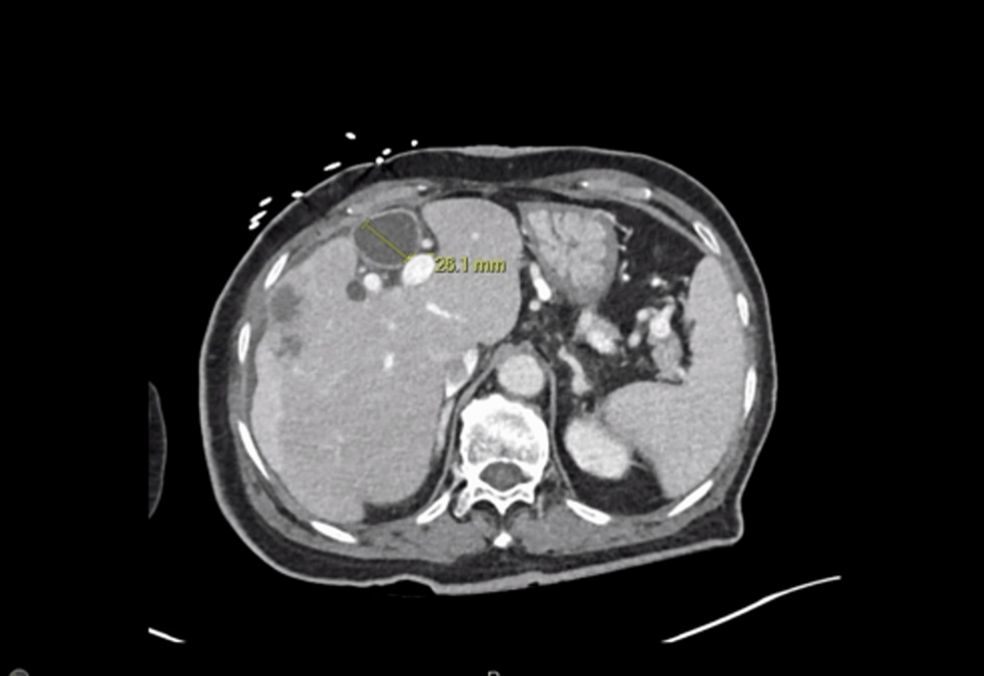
Axial CT of the abdomen with contrast shows dilation of the mid-distal CBD (26 mm). CT: computed tomography; CBD: common bile duct
